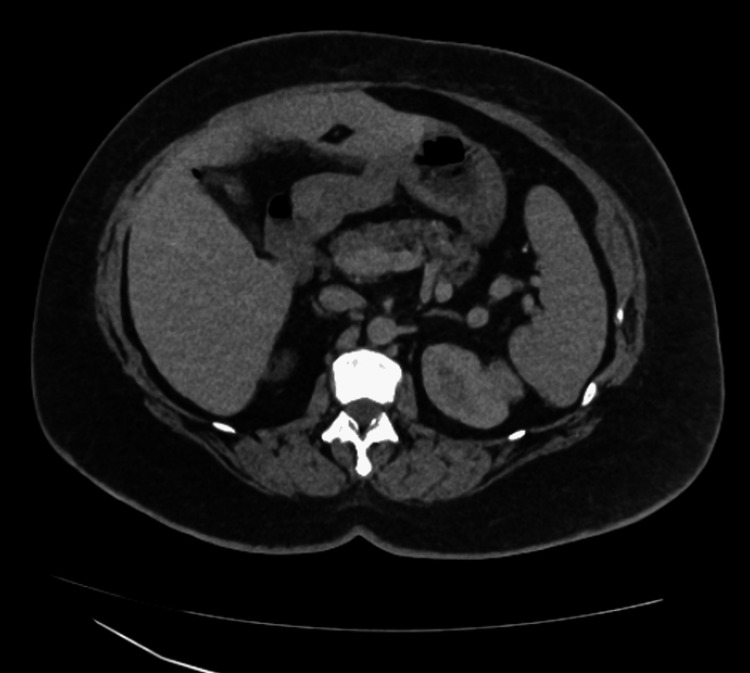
CT abdomen/pelvis (transverse section) showing no evidence of malignancy
